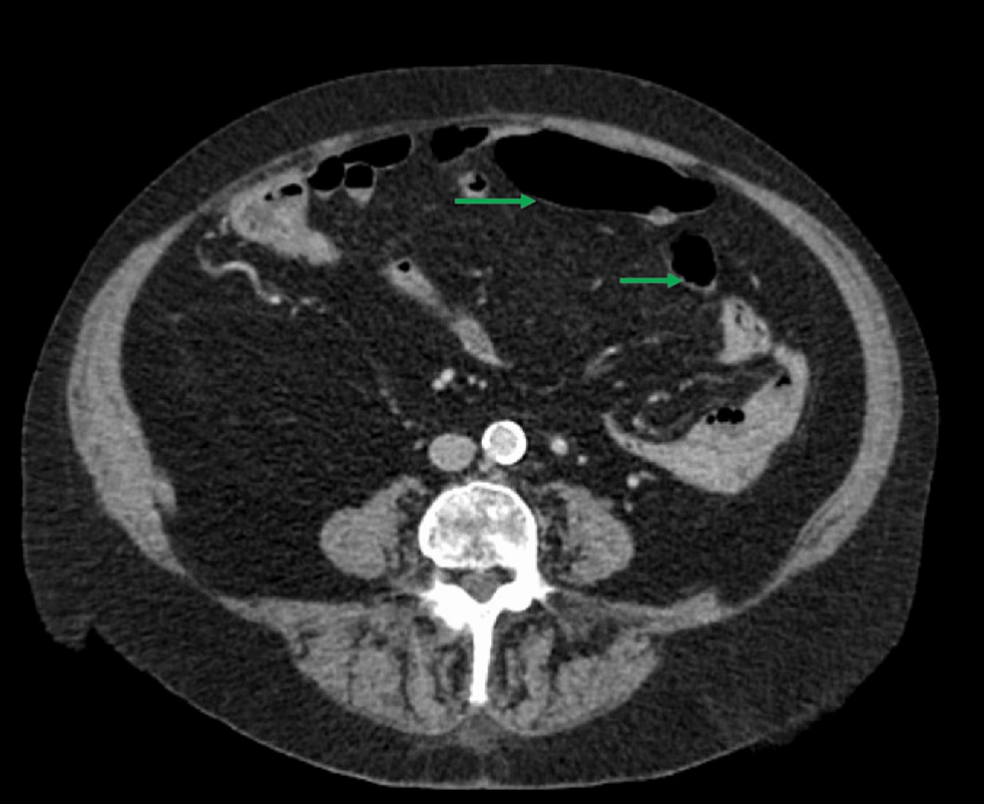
Axial section of CT scan of abdomen and pelvis during hospital admission The green arrows show a normal bowel wall thickness. The presence of mass or fluid collection was ruled out.
